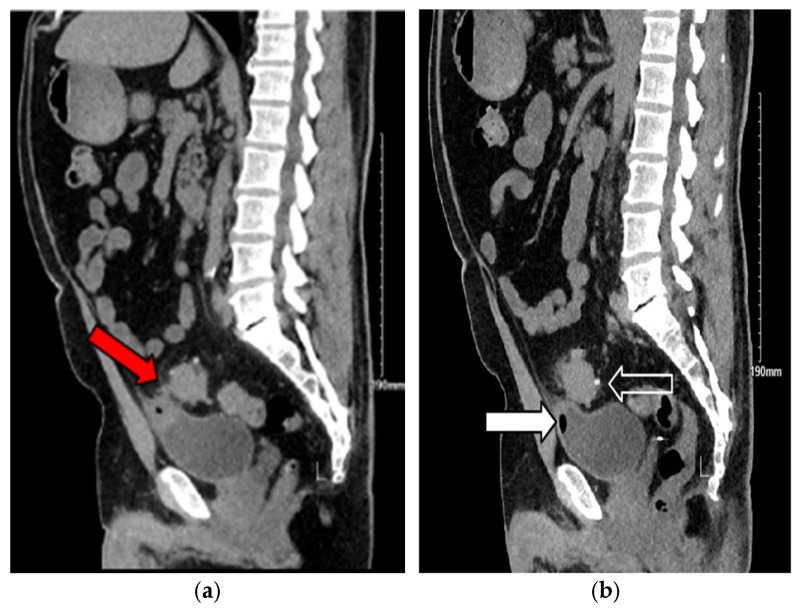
(a) Sagittal noncontrast computed tomography (CT) image showing air within the bladder and sigmoid diverticulitis with a suspected fistulous tract between the sigmoid colon and bladder (red arrow). (b) Sagittal noncontrast CT image demonstrating diverticular outpouching of the sigmoid colon (white outlined arrow) and presence of air within the bladder (white arrow), consistent with colovesical fistula.
